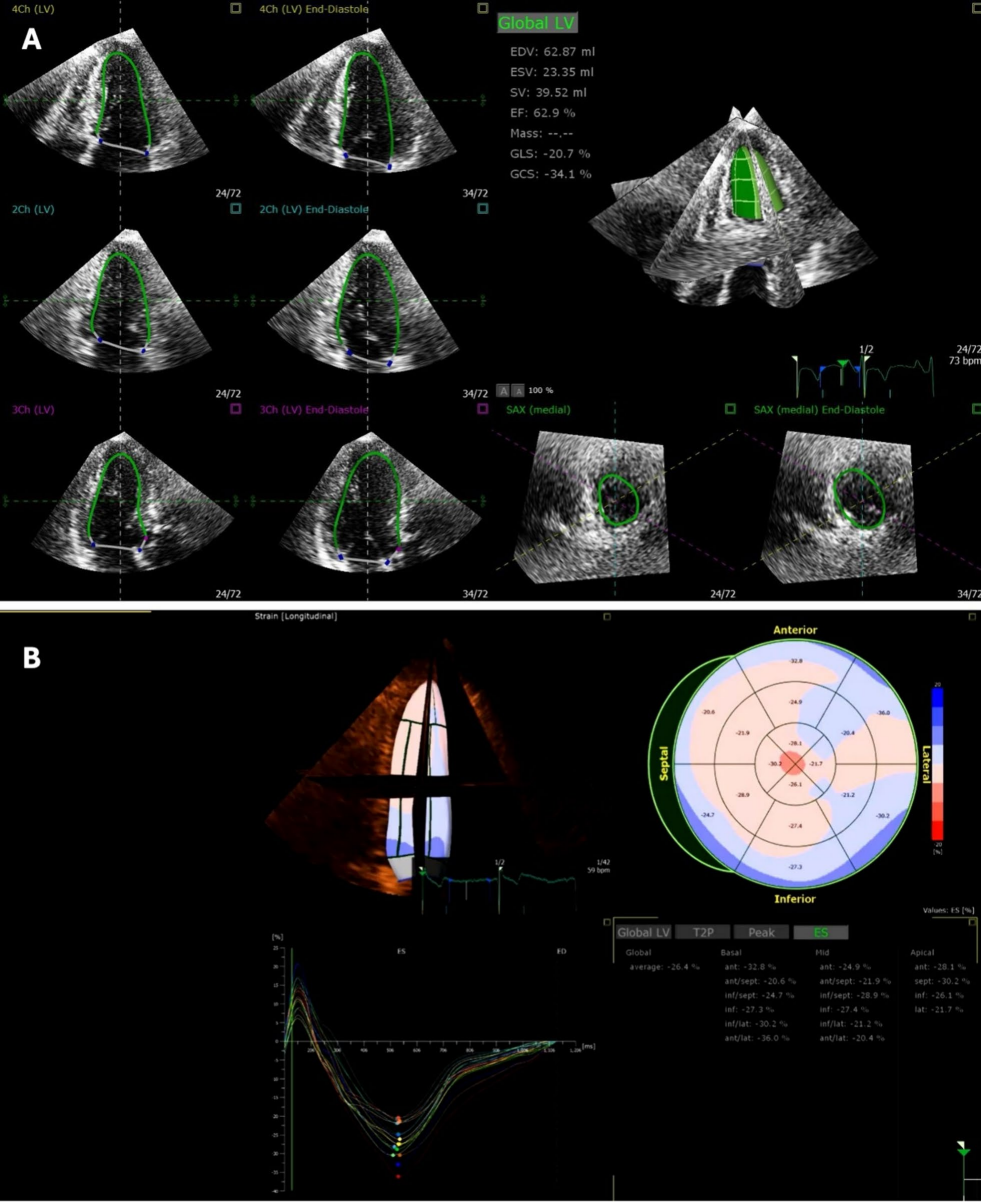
Three-dimensional strain acquisition and analysis. In Panel A, automatic tracking of the endocardial and epicardial contours in the end-systolic and end-diastolic 3D frames. Panel B shows the 3-dimensional echocardiography longitudinal strain results
